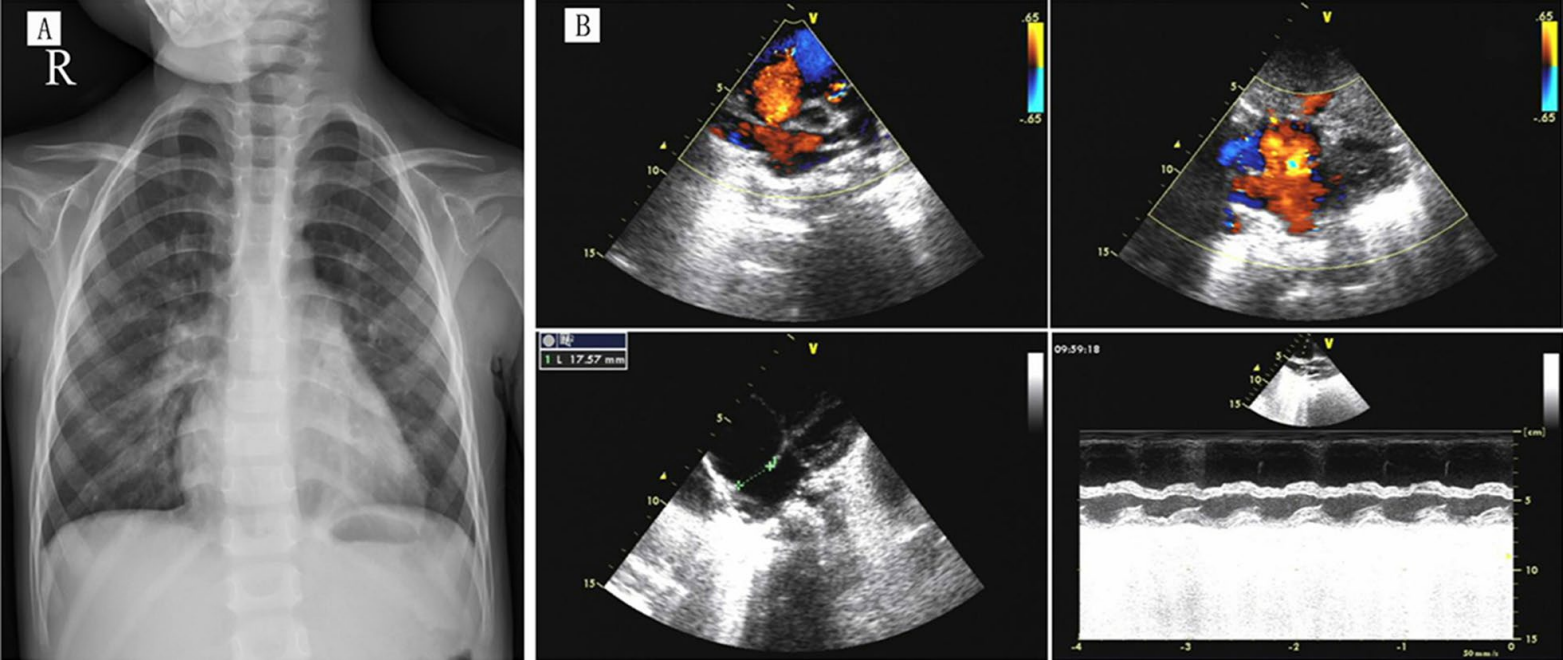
A The previous chest radiography from 4 years ago showed an enlarged podoid, a cardiothoracic ratio of about 0.52, and a slightly prominent left pulmonary artery. Preoperative echocardiography of the atrial septal defect showed the following two-dimensional measurements: RVOT: 24 mm; AOd: 16 mm; LA: 18 mm; AAO: 16 mm; LVDd: 27 mm; LVDs: 15 mm; IVS: 5 mm; LVPW: 5 mm; RV: 23 mm; MPA: 15 mm; RA: 31 × 37 mm; and HR: 107 b/m. Doppler measurements were AV: 1.04 m/s; MVe: 0.88 m/s; MVa: 0.53 m/s; PV: 1.32 m/s; TR: 2.7 m/s; and PG: 29 mmHg. B The diagnoses based on the ultrasound results was congenital heart disease, atrial septal defect (secondary foramen, central type), atrial horizontal left-to-right shunt, and tricuspid regurgitation (mild)
